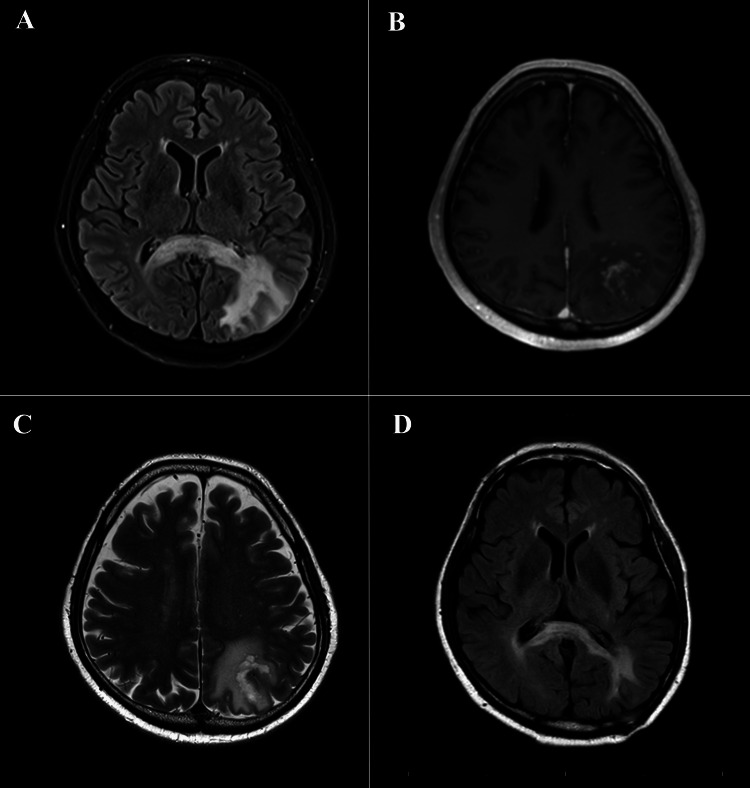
MRI findings (A) Fluid-attenuated inversion recovery, (B) gadolinium (Gd)-enhanced T1-weighted MRI, and (C) T2-weighted MRI at the onset of PML onset. (D) Gd-enhanced T1-weighted MRI after nine months of therapy with mefloquine and mirtazapine
